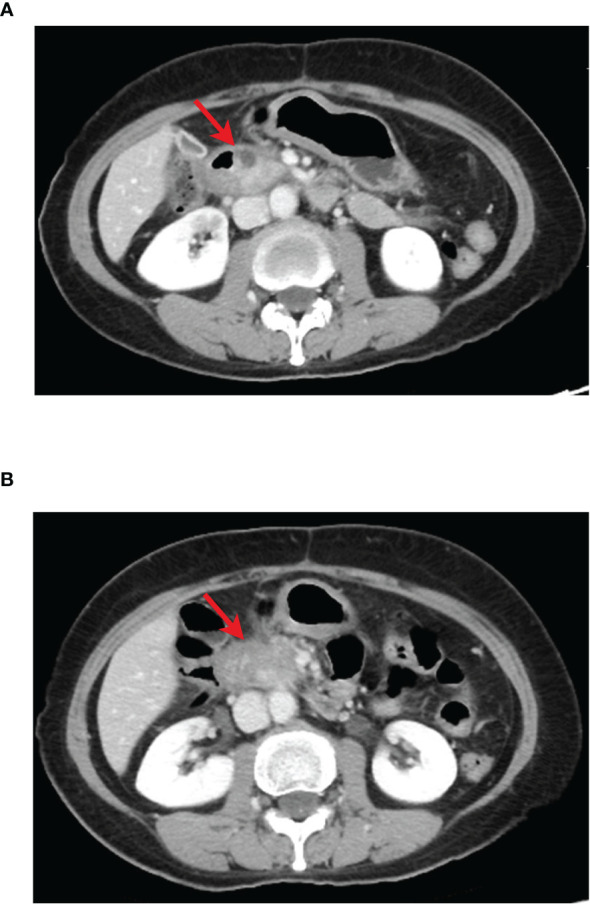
CT images of pancreatic cancer lesion during first-line treatment, red arrow indicates pancreatic lesion. (A) The results of CT reexamination 3 months after the end of sequential chemotherapy showed a slightly enlarged pancreas head mass, prior to AG regimen treatment. (B) The results of CT after two cycles of AG regimen treatment showed significantly enlarged pancreatic head mass compared with that before treatment.
